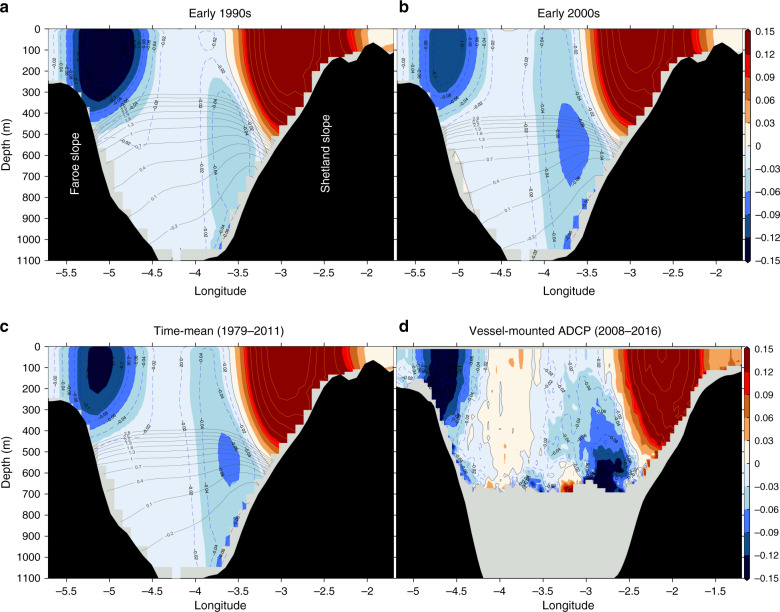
Capturing the deep eastern jet in the FSC. Modeled velocities (southwards/northwards correspond to red/blue shading) across the Faroe–Shetland Channel (FSC) (Supplementary Fig. 1, inset) averaged for a the early 1990s, b the early 2000s, and c 1979–2011 period (model climatology). The gray lines show the corresponding isotherms but only less than 2.8 °C (black shading is bottom topography). d The observed 2008–2016 velocities from the vessel-mounted Acoustic Doppler Current Profiler (ADCP, cf. Fig. 1b). Missing data below 600 m is indicated by light gray. The Faroe–Shetland Channel jet (FSCJ) is banked against the eastern slope as indicated by the sloping isotherms (thin gray lines). It is also strongly bottom-intensified and has another a core at intermediate depths. The overall structure of the FSCJ is similar between the modeled mean velocity and that based on the 9-year long ADCP data (2008–2016) from Norröna (see Methods section).
